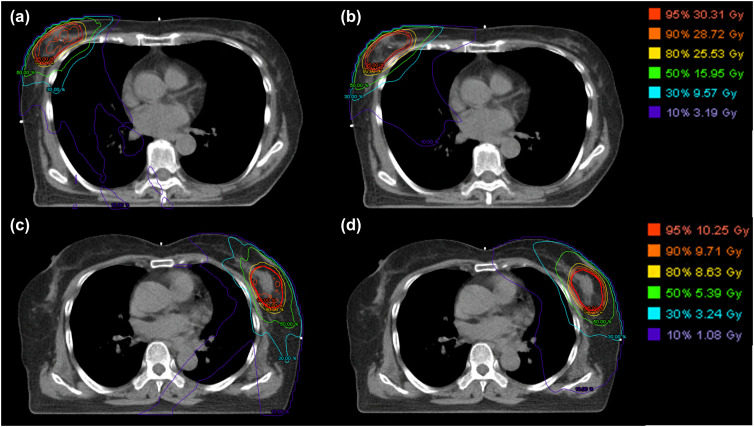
Comparison of dose distributions between the MR-guided S&S IMRT and CT-guided VMAT plans. (a) MR-guided S&S IMRT plan for the 30 Gy PBI treatment of Patient 1. (b) CT-guided VMAT plan for the 30 Gy PBI treatment of Patient 1. (c) MR-guided S&S IMRT plan for the 10 Gy boost treatment of Patient 18. (d) CT-guided VMAT plan for the 10 Gy boost treatment of Patient 18.
